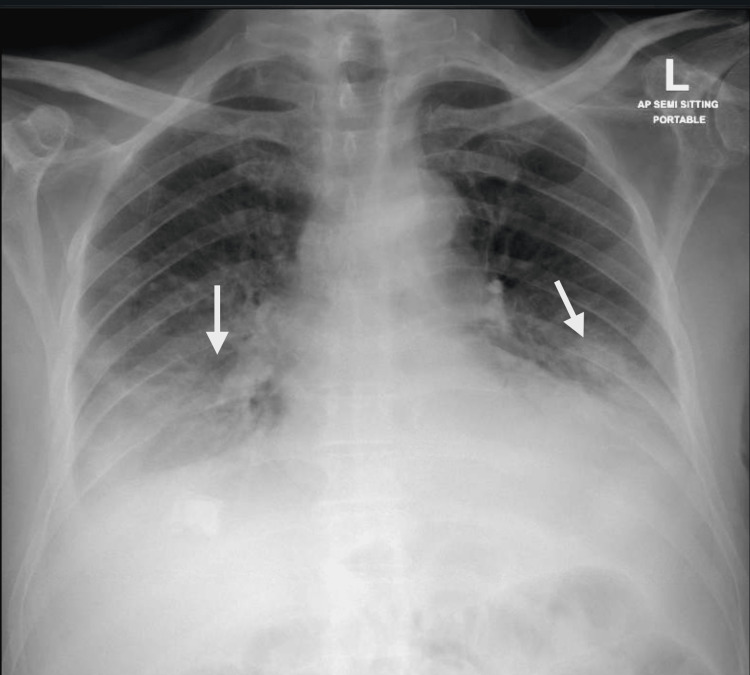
Chest X-ray showing the septal haziness (white arrows)
